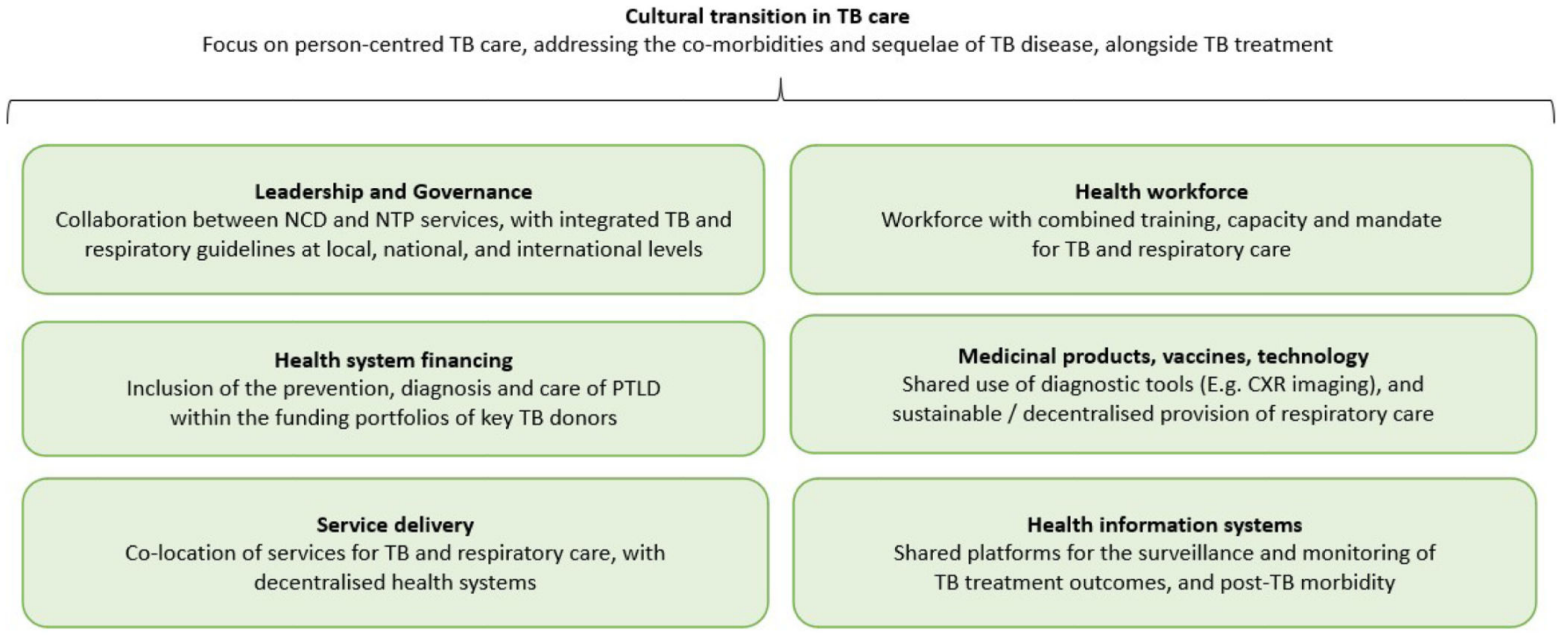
Changes required to the Health System pillars, to delivery PTLD prevention, diagnosis and care PTLD – Post-TB lung disease; NCD – Non-communicable disease; NTP – National TB Programme; CXR – Chest radiograph
